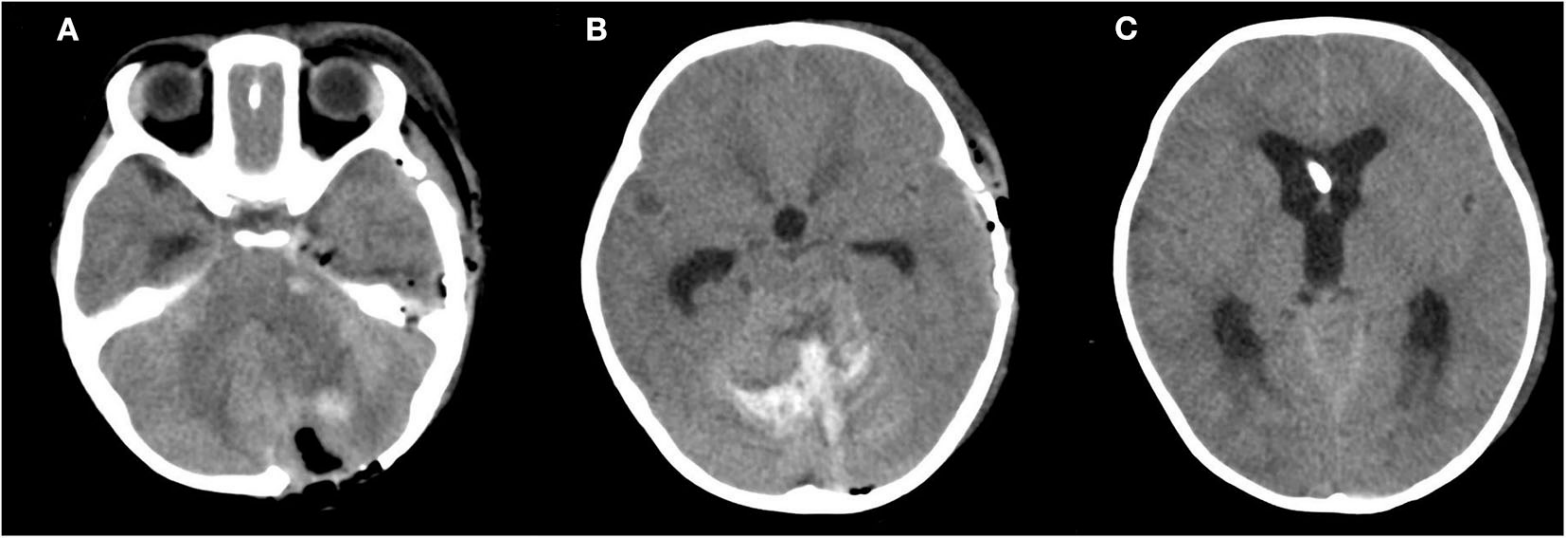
Postoperative computed tomography (CT). (A,B) CT scan revealing postoperative changes and high-density shade in the areas of the cavernous sinus region and cerebellum. (C) A high-density focus in the lateral ventricle.
